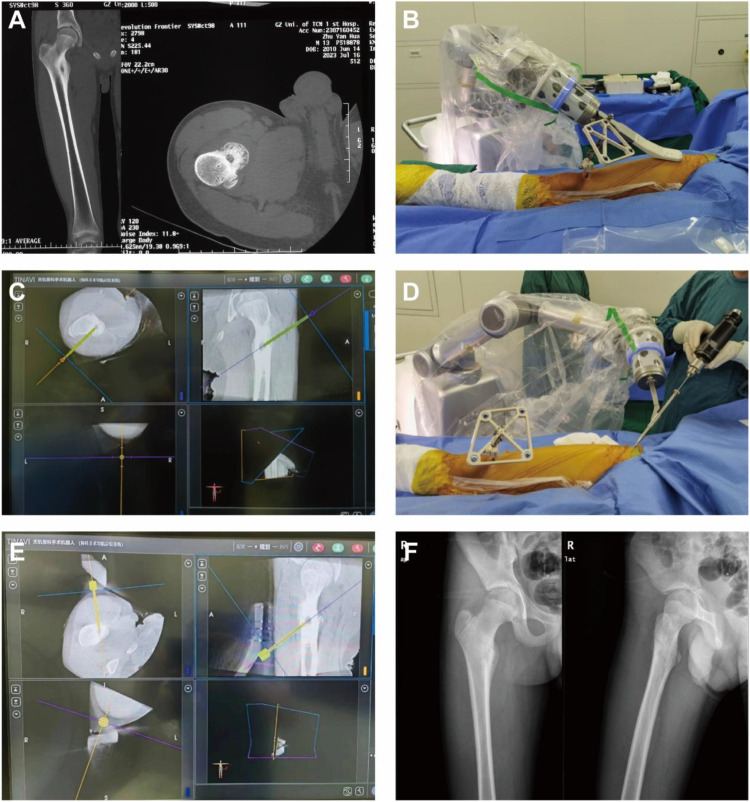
Sequential steps of robot-assisted RFA. The procedure began with (A) a preoperative CT scan identifying the OO, followed by (B) intraoperative localization and scanning. Subsequently, (C) 3D modeling and trajectory planning were performed, guiding (D) the insertion of the guide needle. (E) Radiofrequency ablation was then applied. (F) A follow-up radiograph confirmed the treatment outcome.
